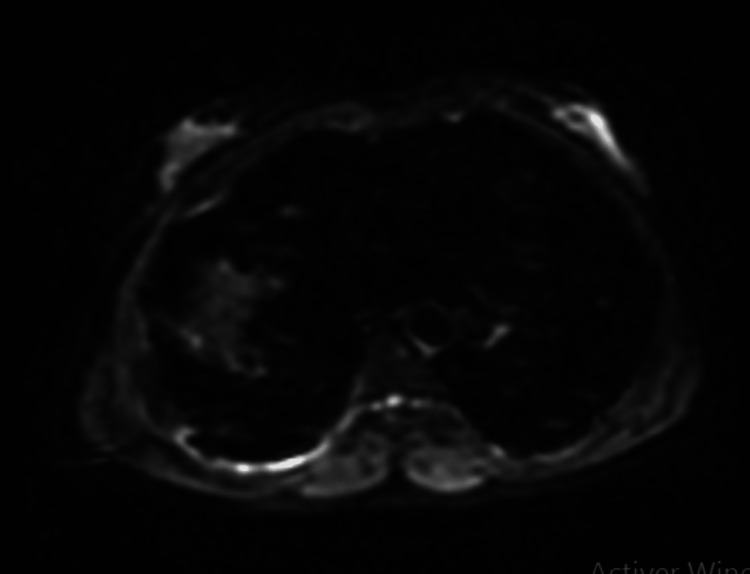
Thoracic MRI: diffusion sequence The lesion does not show diffusion restriction MRI: magnetic resonance imaging
